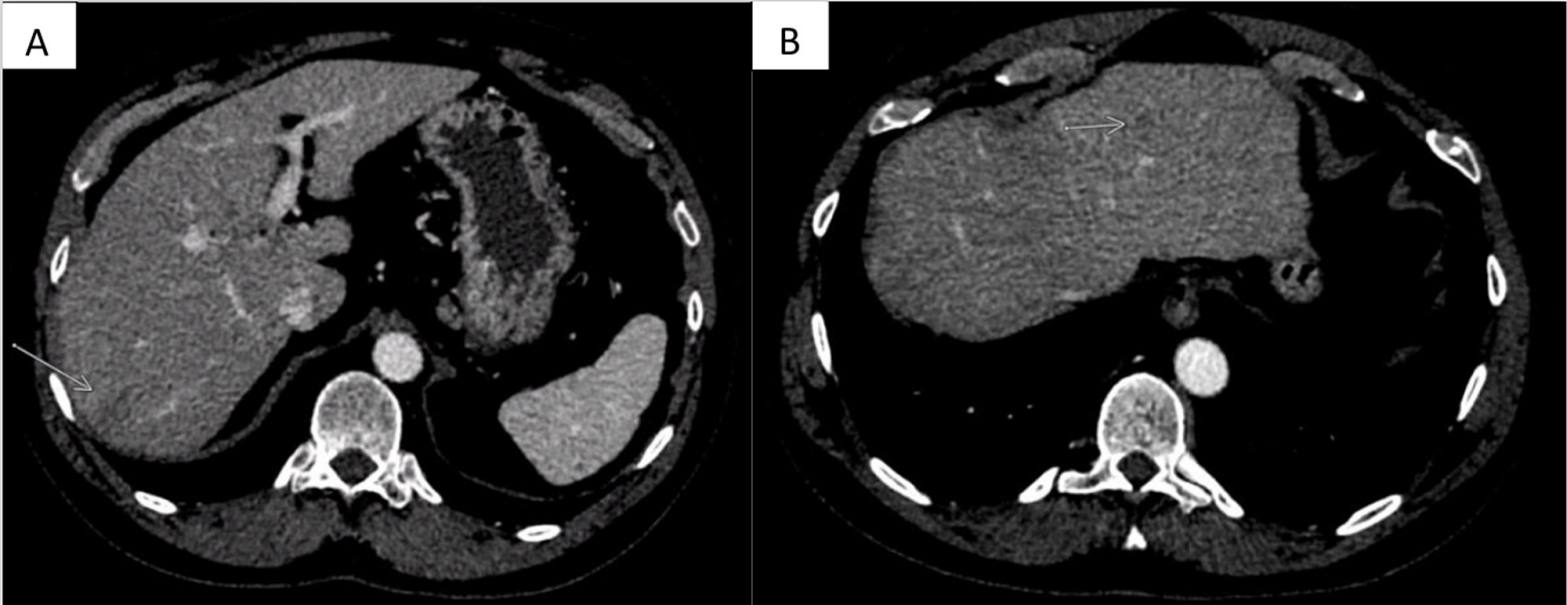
One-year post-treatment follow up CT images Axial post-contrast CT through the liver shows that the right hepatic lobe mass has decreased in size and conspicuity (arrow in A). The left hepatic lobe mass has also decreased in size and is now barely visible (arrow in B).
